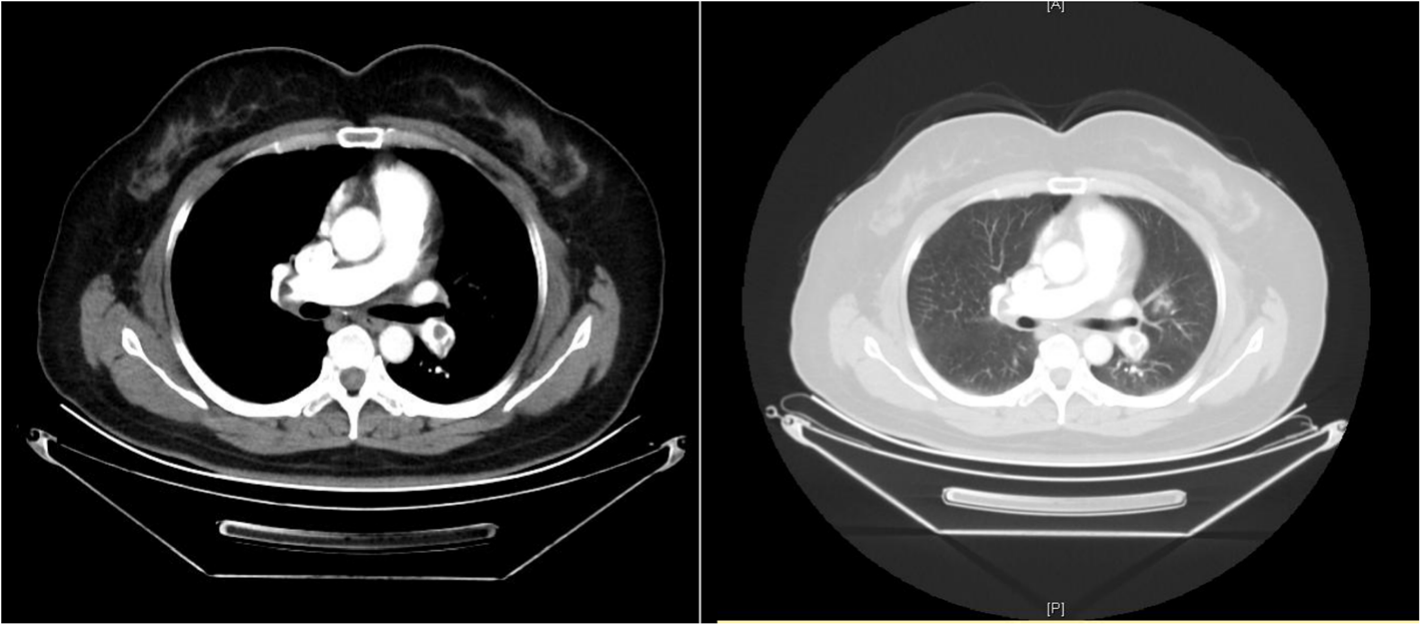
Computed tomography pulmonary angiography (CTPA) revealing filling defects in the main pulmonary artery and upper and lower pulmonary artery branch
